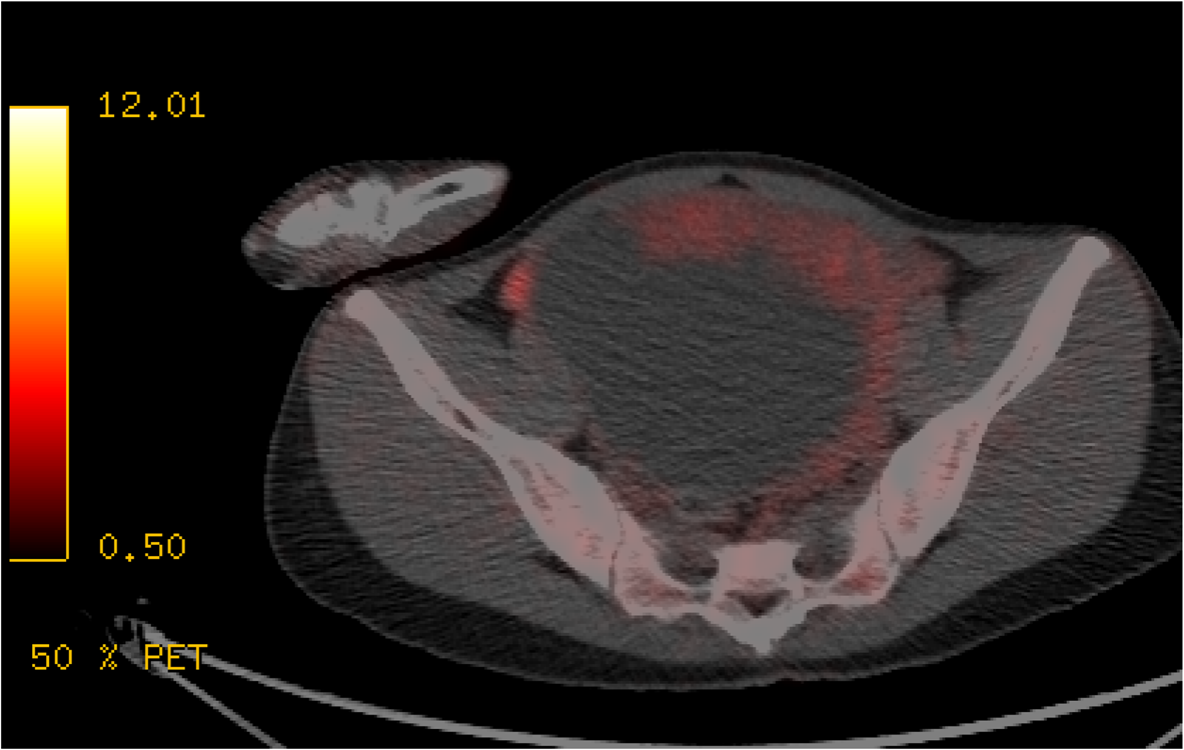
PET-CT shows mild FDG uptake in solid components of the tumor. (SUV: 2.11)
